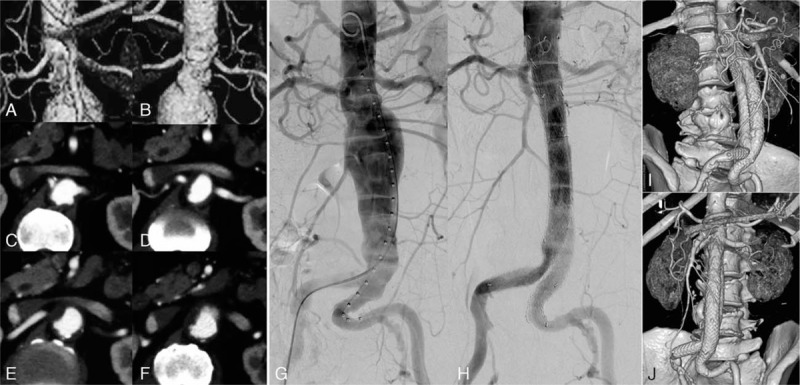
Preoperative CTA reconstruction (A, B) revealed an abdominal aortic aneurysm with hostile neck anatomy. Continuous cross-section scanning (C–F) revealed an aneurismal neck mural thrombus covering 50% of the circumference and an imminent rupture of the aneurysm in the aneurismal neck area (F). Intraoperative DSA (G, H) revealed that the abdominal aortic aneurysm was well repaired with a fenestrated stent graft. The 3 target arterial branches were reconstructed using a balloon-expandable stent (Scuba, InvatecS.r.l., Italy) for the superior mesenteric artery, and 2 stent grafts (Viabahn; W.L. Gore & Associates, Inc., Flagstaff, AZ) were used for the reconstruction of the bilateral renal arteries. The CTA follow-up at 6 months after surgery (I, J) showed smooth flow in the target arteries, with no aneurysm endoleaks. CTA = computed tomographic angiography, DSA = digital subtraction angiography.
